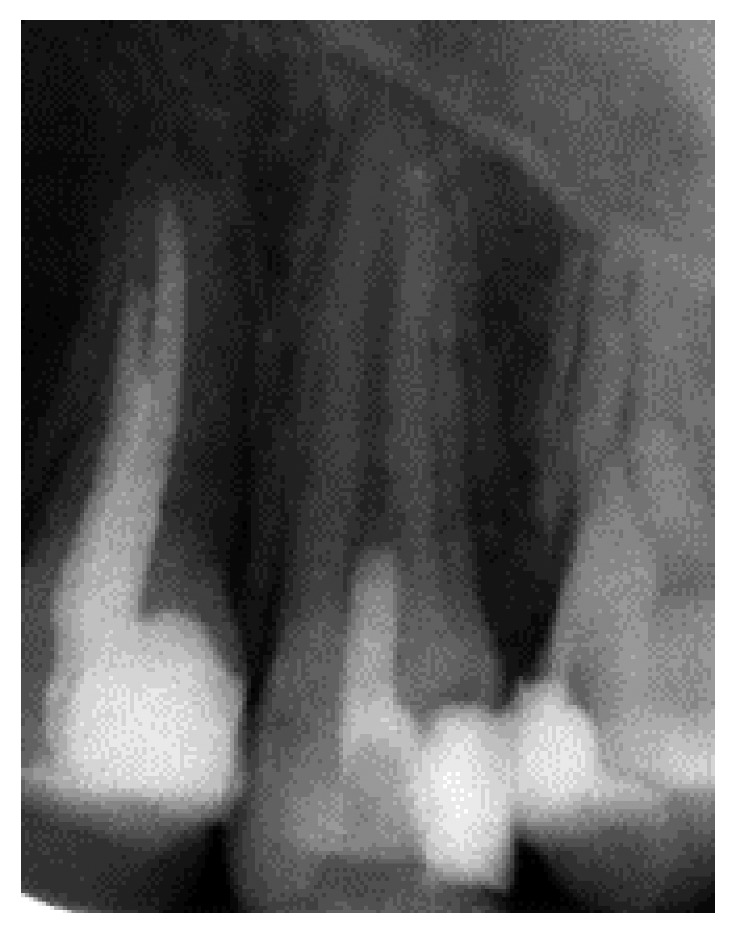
Postoperative radiograph presenting placement of the MTA and glass ionomer cement.
